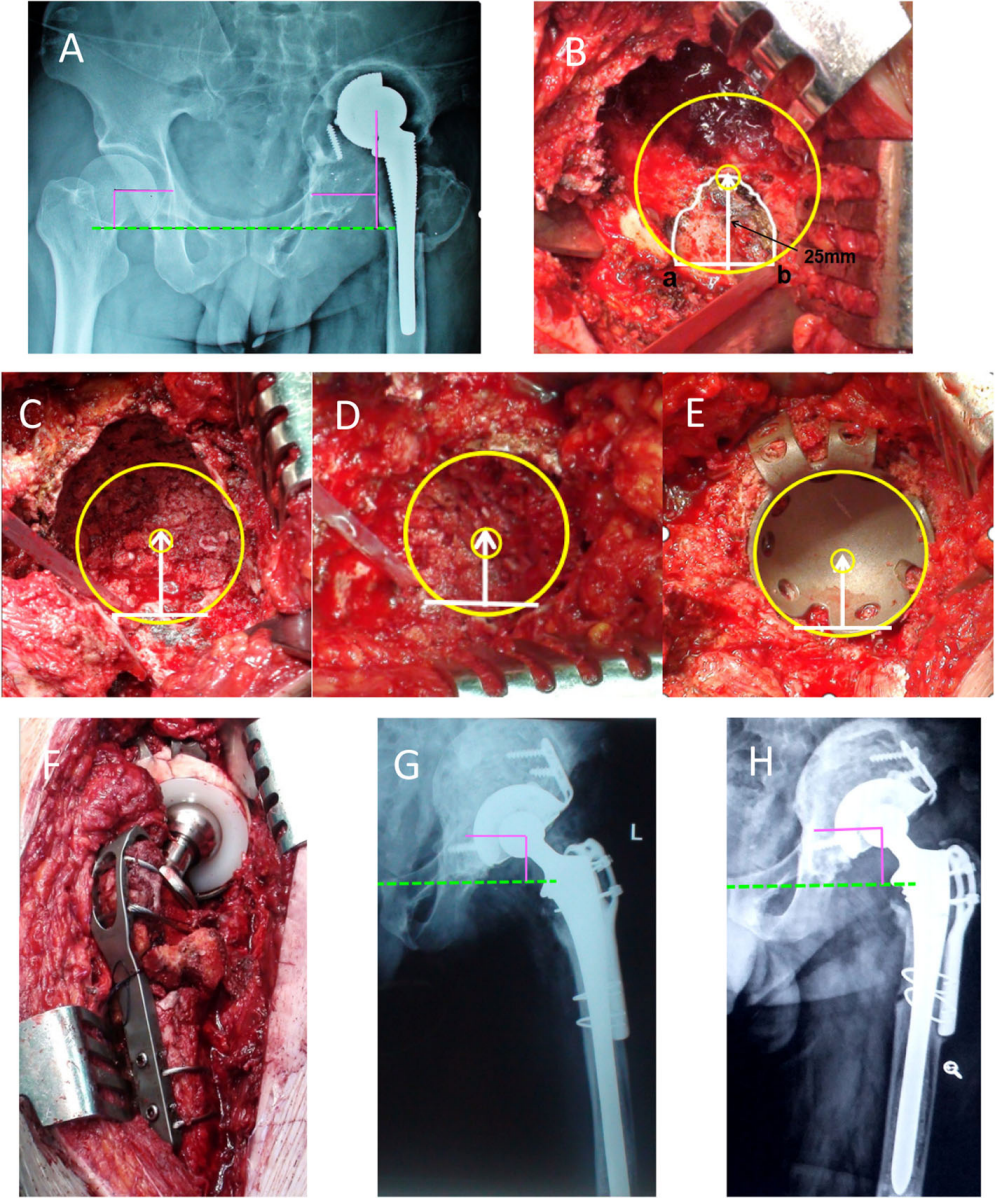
A 54-year-old male patient with aseptic prosthesis loosening 17 years after THA. a Preoperative x-ray showed paprosky type IIIB acetabular bone defect in left hip. b Intraoperative image showed remnant structures of the Harris fossa and acetabular notches. a, b point represented the remnant of acetabular notches. The white curve represented the remnant of Harris fossa. The acetabular center was located at 25 mm above the perpendicular bisector of acetabular anterior and posterior notch line. c Sclerous acetabular bone bed was concentrically reamed using the above located point as the center of circles. Impaction bone graft was used to reshape the acetabulum. d New acetabulum was reconstructed by impaction bone graft. e The acetabular enhancement cup was installed. f Median gluteal muscle insertion site was reconstructed, and the proximal femur was reconstructed by structural bone graft. g Postoperative x-ray showed hip rotation center was restored. h Ten years follow-up x-ray showed vertical and horizontal acetabular migration were 3.6 mm and 2.8 mm respectively
